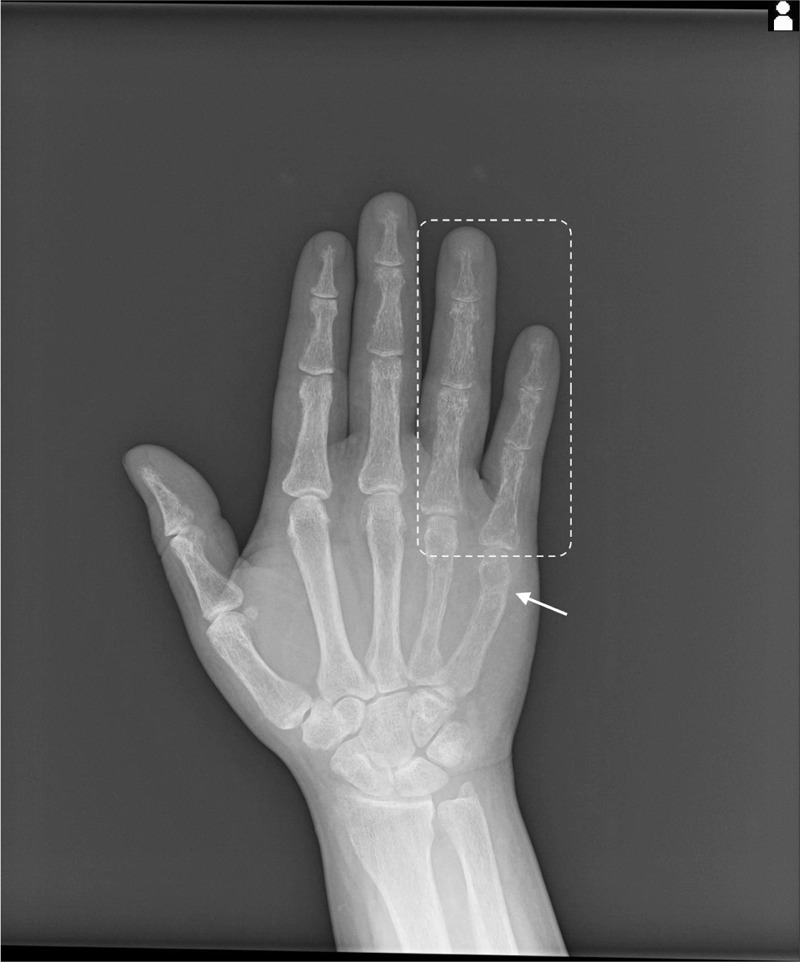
An x-ray showing the fracture (arrow) and cortex bone erosion of right fourth and fifth finger (surrounded area) 2 weeks following the injury.
